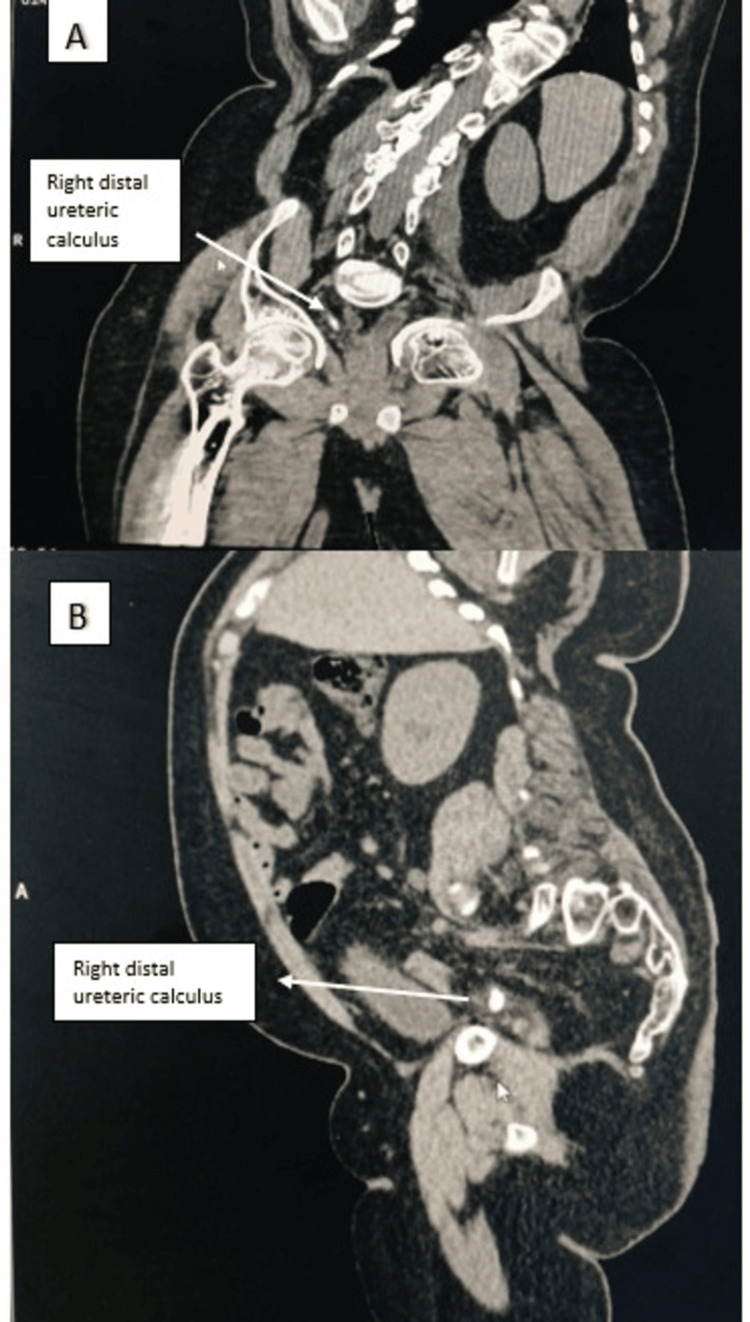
CT KUB images demonstrating an impacted distal ureteric calculus. CT images (coronal and sagittal) showing a right distal ureteric calculus indicated by the white arrow.
